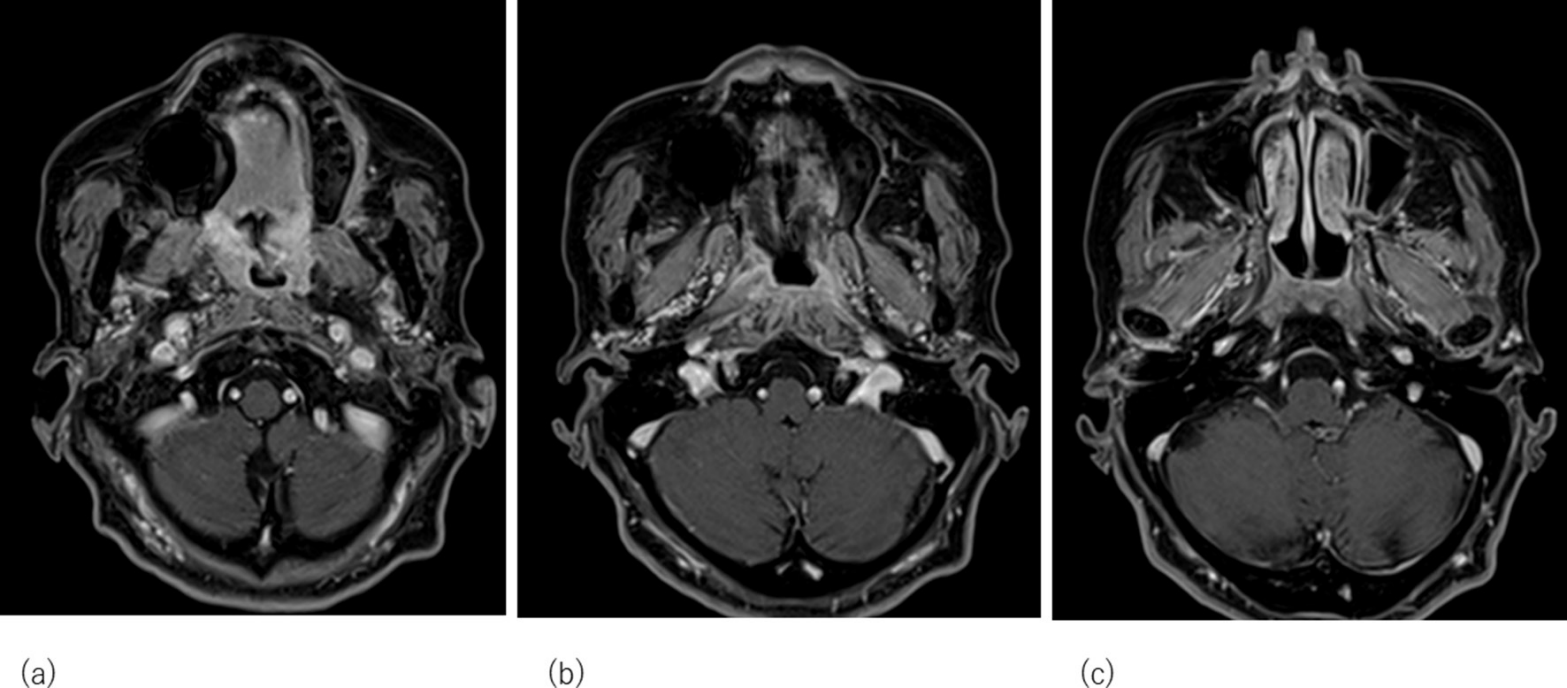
Magnetic resonance image taken 5 months after interstitial brachytherapy. No evident residual tumour was found in the nasopharynx.
